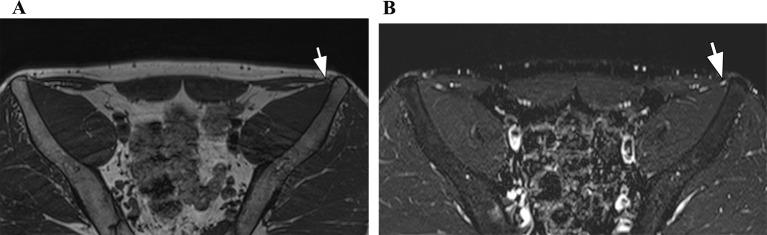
Lateral femoral cutaneous nerve injury. 49 year-old male with left meralgia paraesthetica. Axial 3D volumetric T1-weighted (A) and STIR (B) images of the pelvis show the left lateral femoral cutaneous nerve with thickening and increased signal (arrows), indicating neuropathy
